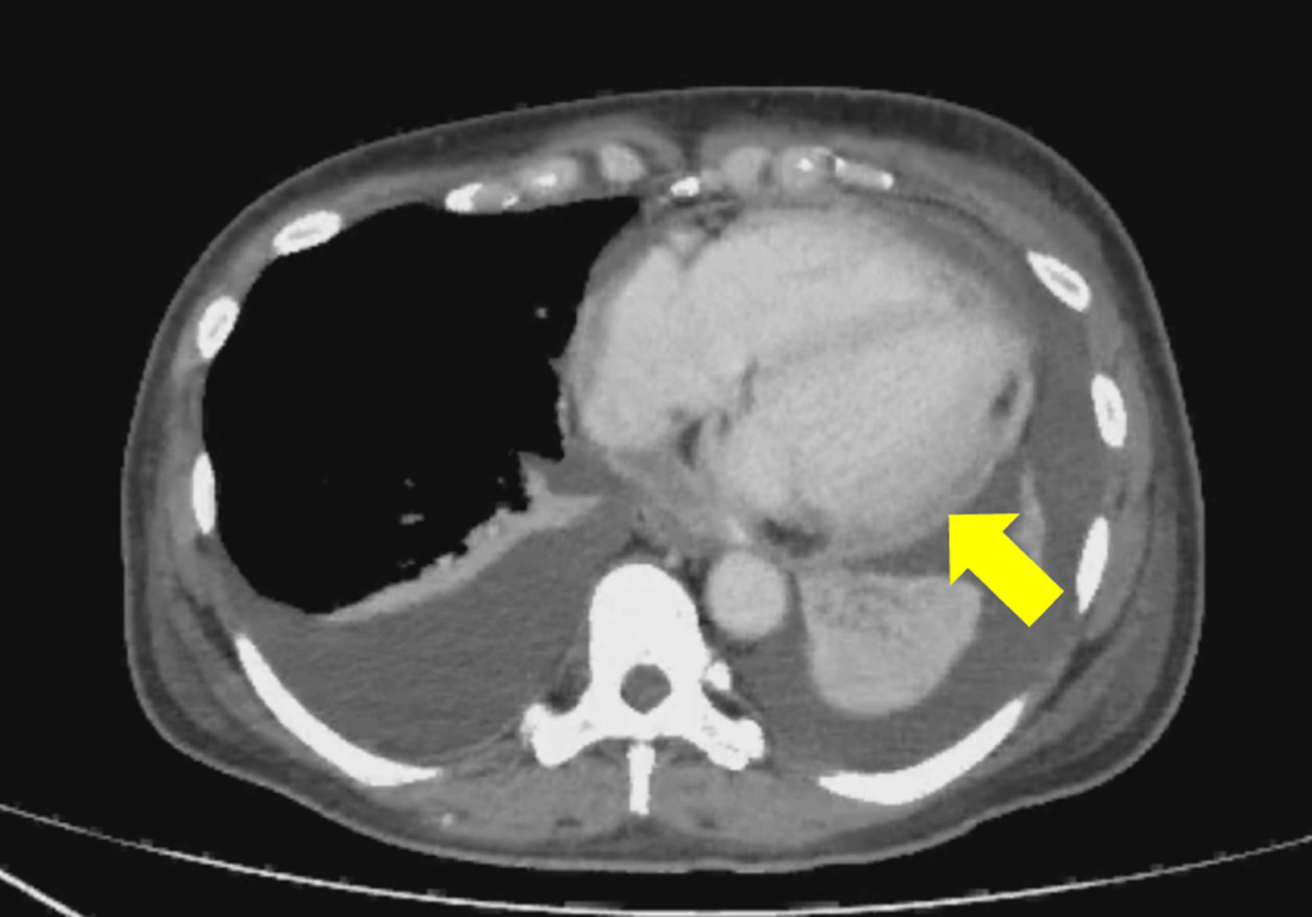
Contrast-enhanced chest and abdominal computed tomography The findings included pericardial thickening (arrow), a small pericardial effusion, bilateral pleural effusions, inferior vena cava dilation, and hepatic congestion.
